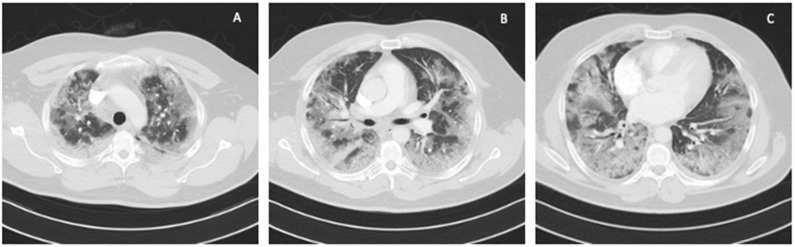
Chest CT scan on admission to the ICU: (A) upper lung segments; (B) middle lung segments; (C) basal lung segments (Case 1).
